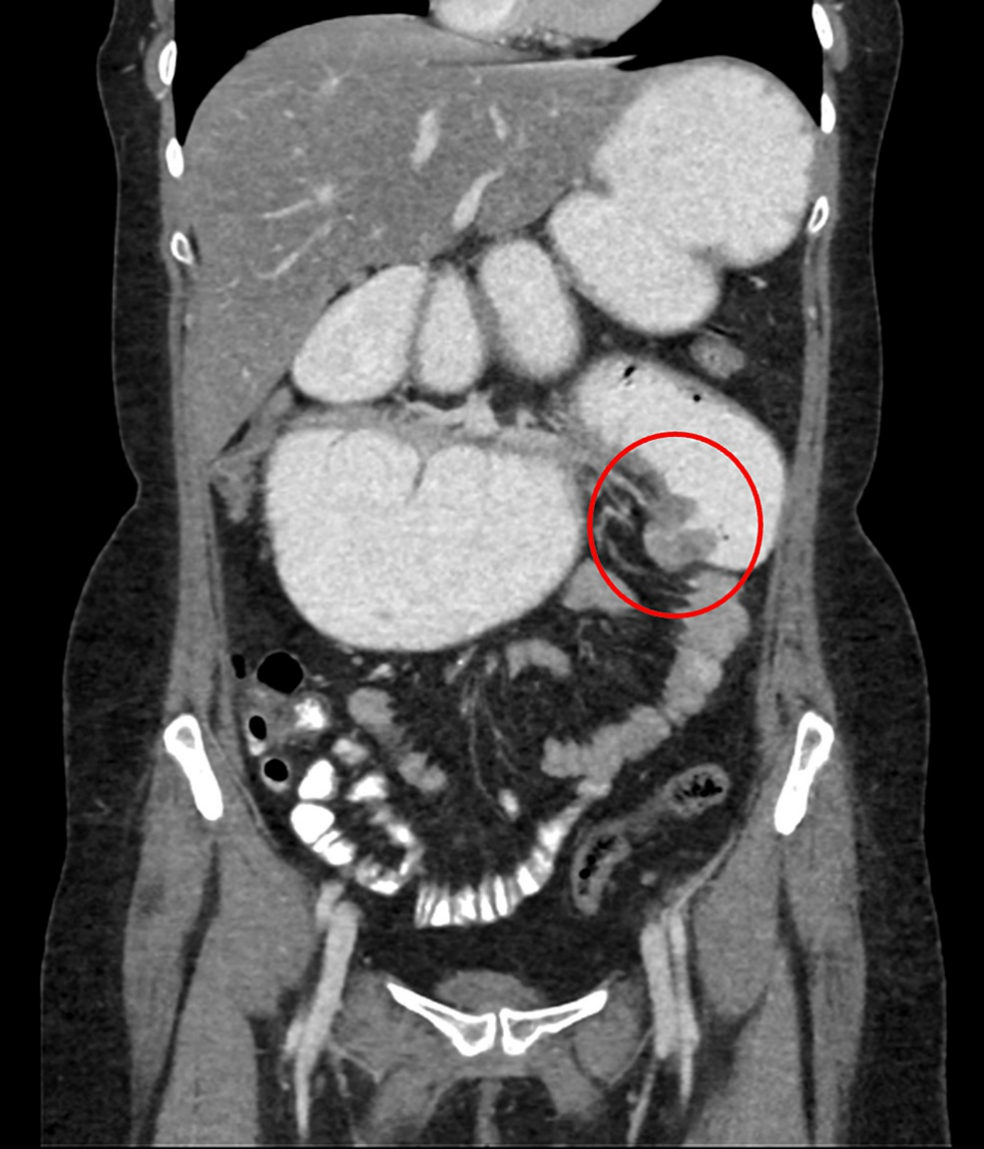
Coronal computed tomography scan showing shouldering of the mass associated with significant dilatation of the proximal jejunum, duodenum, and stomach.
